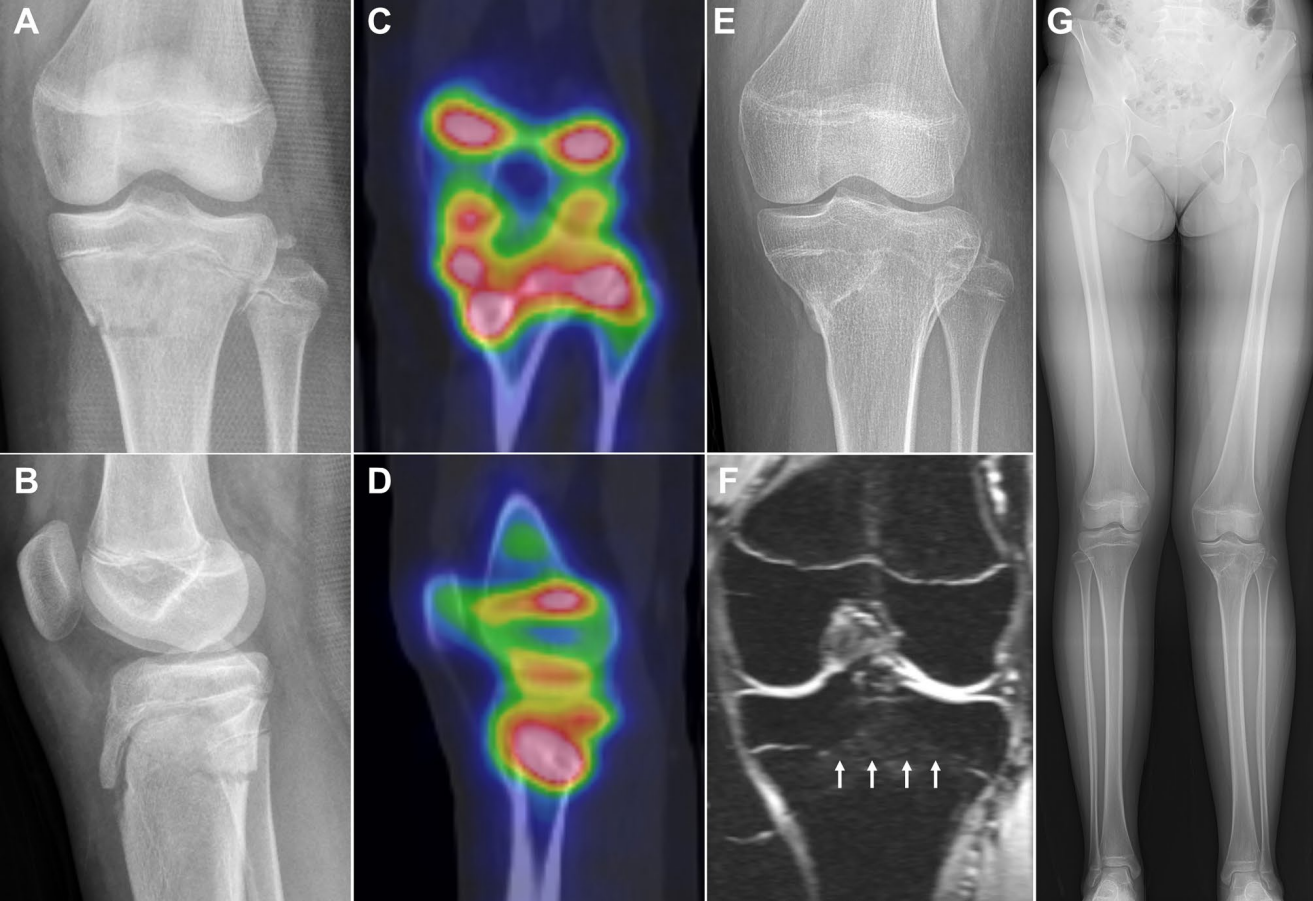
A case of a girl demonstrating insufficiency of bone SPECT/CT in selecting a treatment plan. She had a left proximal tibial physeal fracture at the age of 10.8 years (A and B). Bone SPECT/CT performed at post-operative 6 months reveals hyperactivity of the overall proximal tibial physis, contrary to our expectation of hypoactivity in some areas of the physis (C and D). Growth disturbance is suspected on knee radiograph at post-operative 10 month (E). She underwent permanent epiphysiodesis of the proximal tibia and fibula due to extensive bone bridge (arrows) (F) and progressive limb length discrepancy (G). She plans to undergo tibial and fibular lengthening after reaching skeletal maturity
